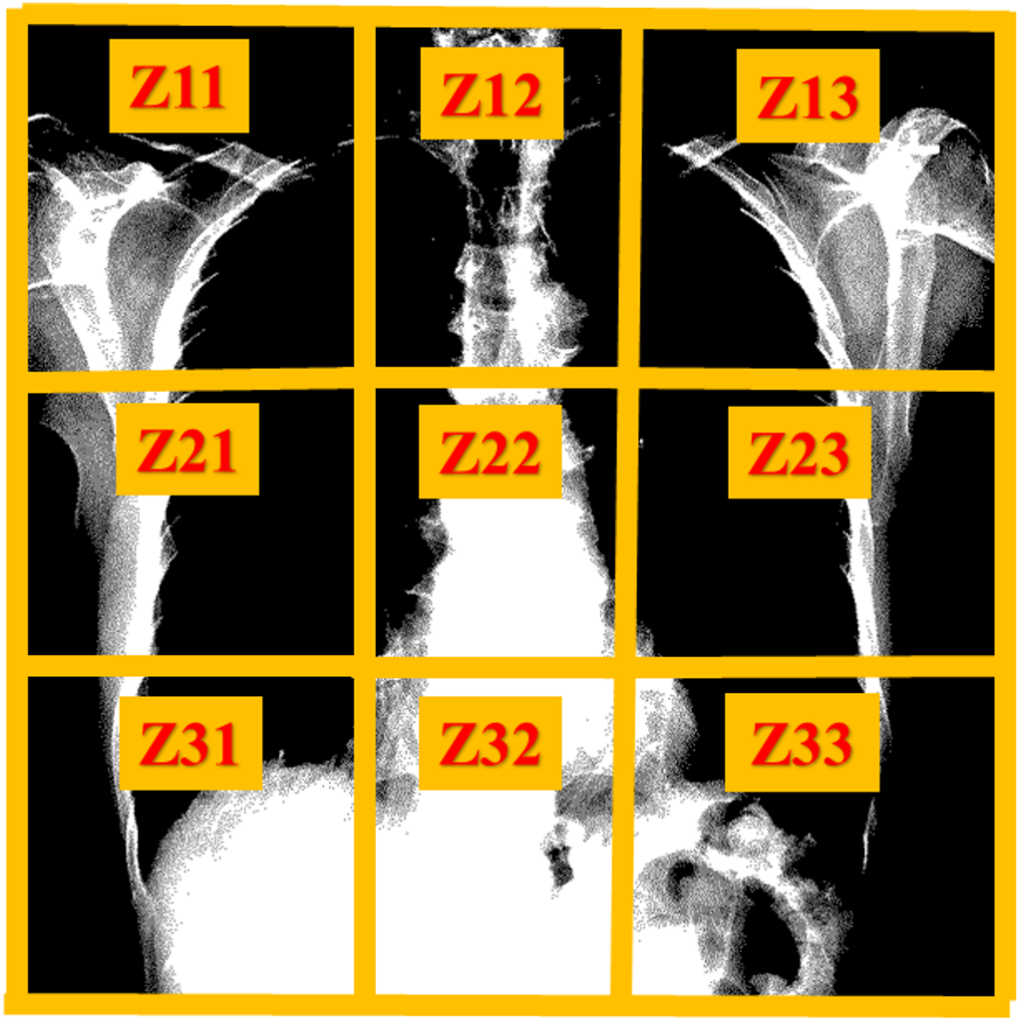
Zones of chest X-ray images for attention visualization.
